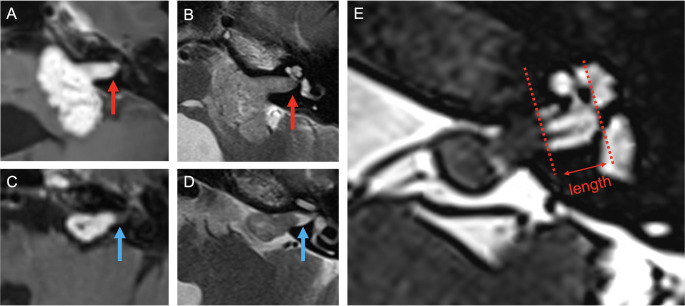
MRI examples of a fundal CSF cap. T1 Post-contrast (A) and T2 (B) axial MRI acquisitions of a patient with NF2 related schwannomatosis with a large left sided vestibular schwannoma. Contrast enhancing tumour is seen all the way to the fundus of the meatus, and there is an absence of high T2 signal (indicating the absence of cerebrospinal fluid) in the fundus of the meatus, suggesting no fundal CSF cap (red arrows). T1 Post-contrast (C) and T2 (D) axial MRI acquisitions of a patient with a sporadic left sided vestibular schwannoma demonstrating a clear separation between the contrast enhancing tumour and the meatal fundus, with high T2 signal in the fundus in keeping with a fundal cap (blue arrows). Fine slice heavily T2 weighted imaging of the same patient (E) can be used to measure the length of this cap
